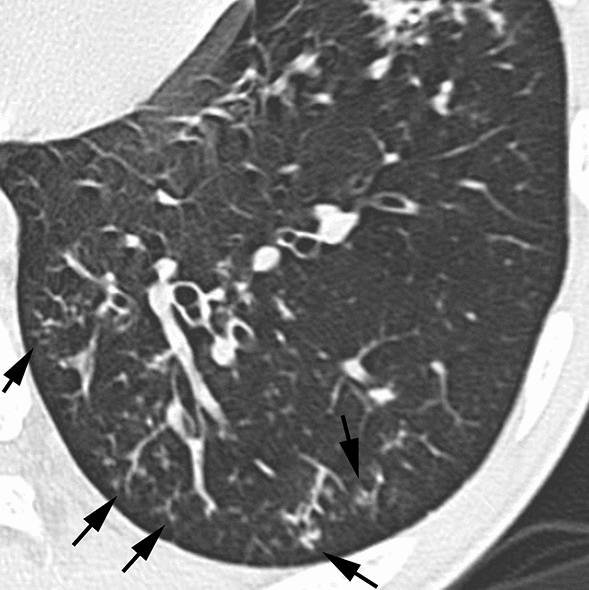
Infectious disease (invasive aspergillosis) in an 18-year-old male patient with acute myelogenous leukemia. HRCT image of the left lower lung showing nodules with a centrilobular distribution and TIB pattern (arrows). Note that ILS thickening is not evident
